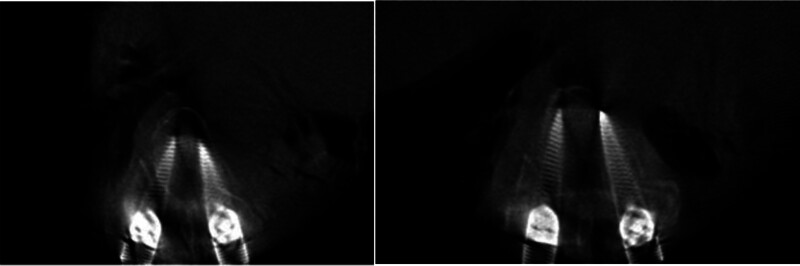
Intraoperative O-arm scanning of thoracic pedicle trajectories demonstrating optimal positioning of T7 and T9 screws. O-arm = O-shaped arm x-ray machine.
